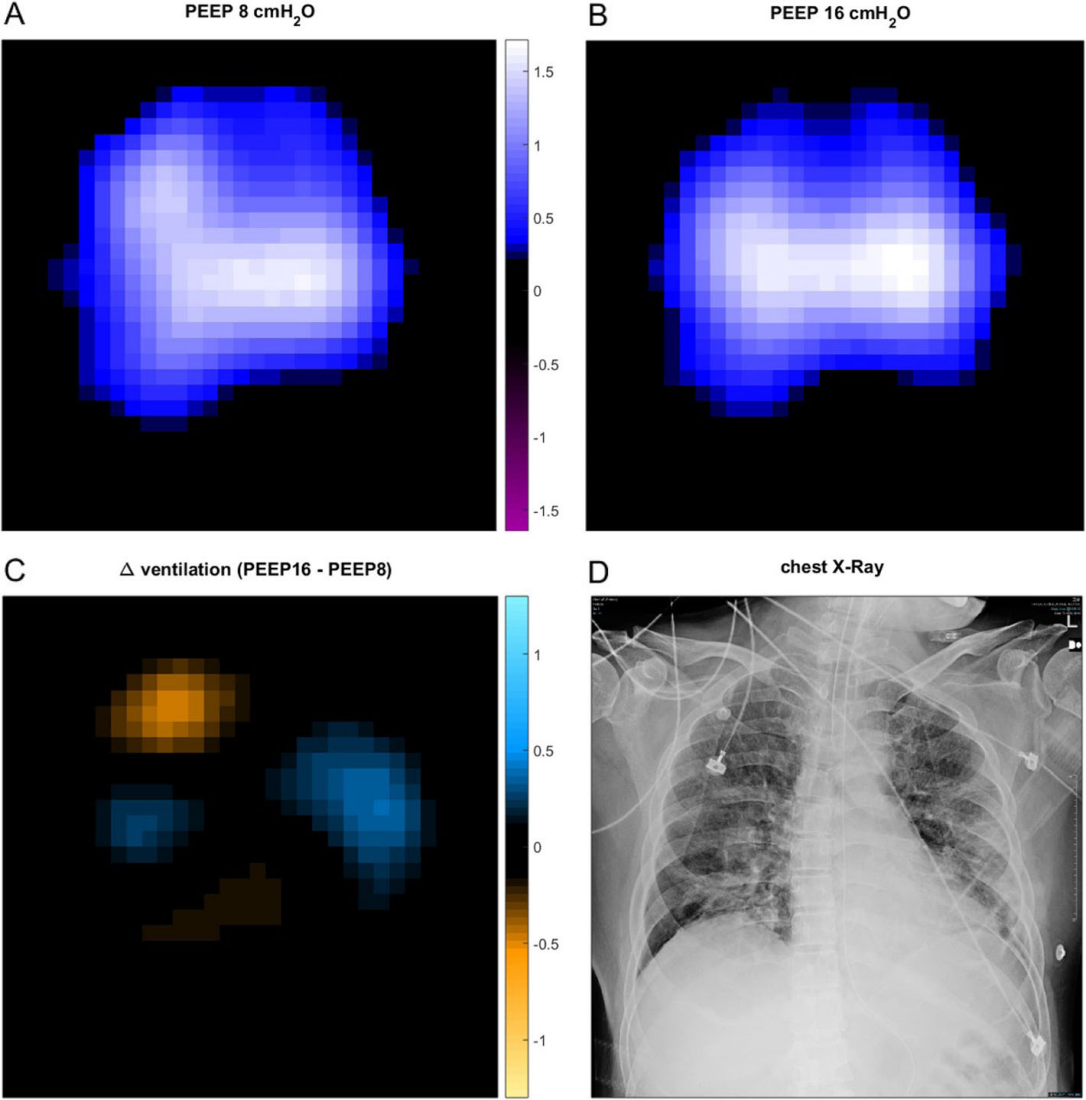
Electrical impedance tomography measurement of a COVID-19 patient during PEEP increases from 8 to 16 cmH_2_O, and the chest X-ray on the same day. a, b Tidal variations show ventilation distribution during tidal breathing. Highly ventilated regions are marked in light blue. c The differences in ventilation distribution between PEEP of 16 and PEEP of 8 cmH_2_O. Ventilation loss is marked in orange whereas ventilation gain is marked in blue. d Chest X-ray shows increased infiltration to the left lower lung field and to the right lower lobe
